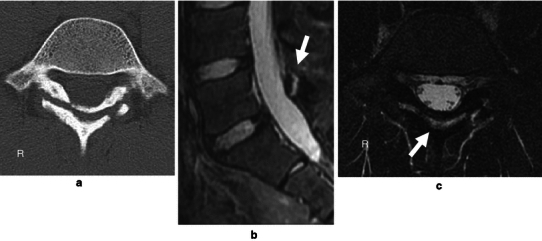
a CT images show a clear defect in the L5 lamina, which is seen intralaminally. b MRI shows signal changes along the fracture line with high intensity on a fat-saturated T2-weighted image
